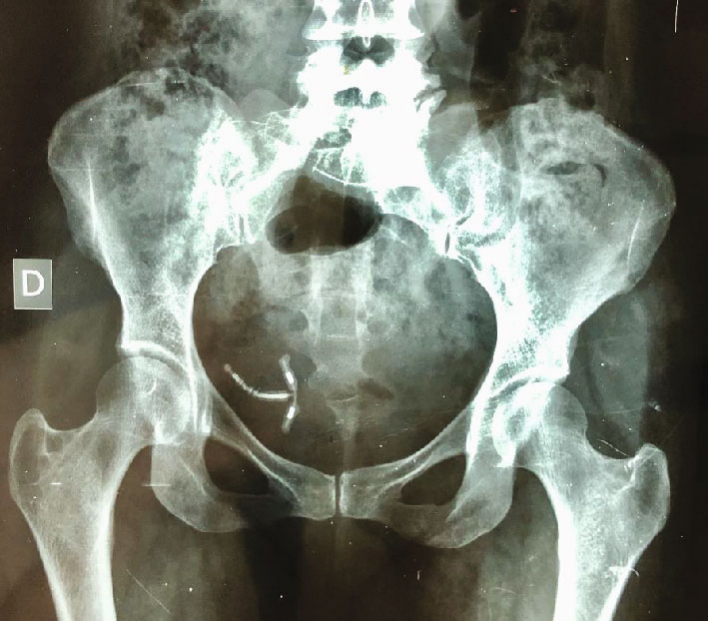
A mislocated IUD in the lower left quadrant of the abdomen showed on the plain abdominal X-ray.
